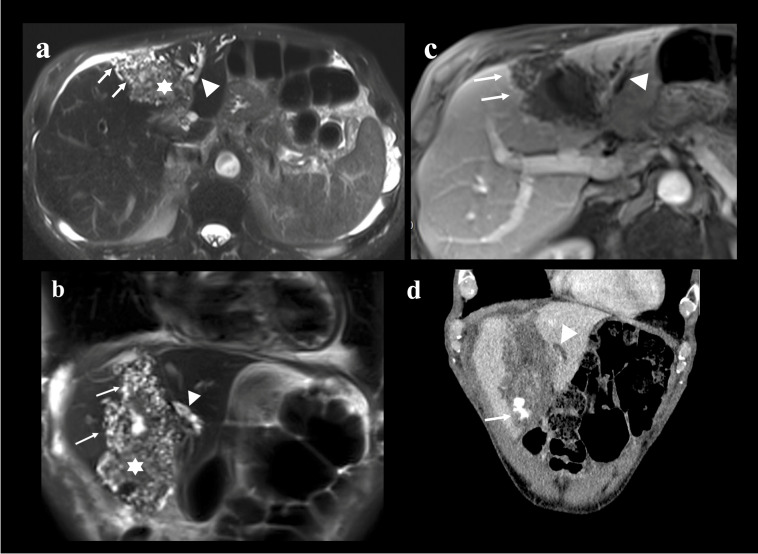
T2-weighted axial (a) and coronal (b) MR images show the characteristic multivesicular structure of alveolar echinococcosis (AE) located in the fourth liver segment detected in a 68-year-old man. The lesion is composed of multiple small cysts surrounding a solid component (a,b, stars) and a large irregular cyst. Note the extension into the left liver lobe with bile duct infiltration and consecutive biliary dilatation (a–d, triangles). The correspondent T1-weighted axial MR image (c) after intravenous gadolinium injection shows the typical faint enhancement at the periphery of the lesion (c, arrows) and the infiltrative character because of ill-defined margins, particularly well seen in the posterior part of this lesion, explaining the frequent misdiagnosis as a tumor. The coronal CT-image of the same patient (d) nicely demonstrates the typical coarse calcification of AE (c, arrow).
